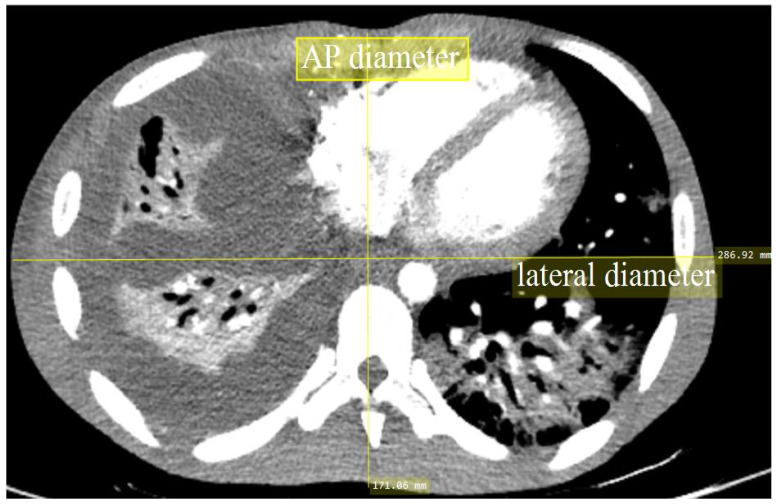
Patient′s size measurement at the mid-slice location of the transverse CT images.
